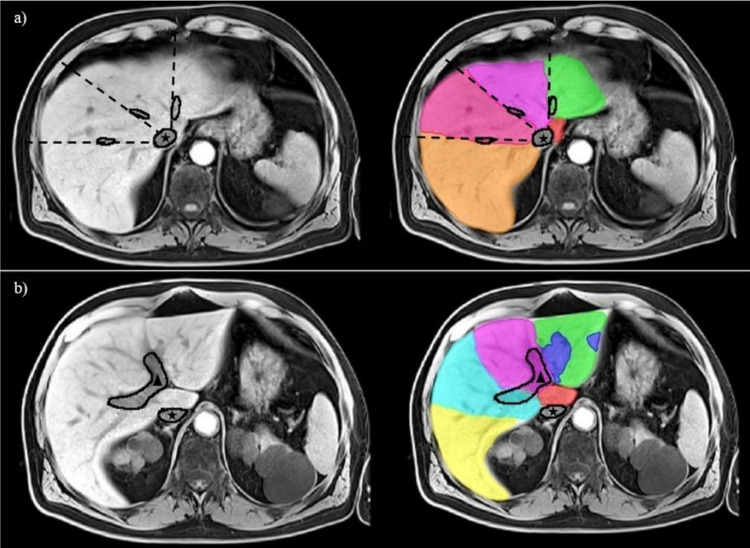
MRI cross-section of the liver at different slice positions: Star indicating the IVC, triangle indicating the bifurcation of the portal vein, crosscut of dotted line through the hepatic veins (plain encircled) to the IVC, delineating the liver segments. Clockwise in a S 7,8,4,2,1—orange, magenta, pink, green, red, and b S 6,5,4,3,2,1—yellow, cyan, pink, blue, green, red
